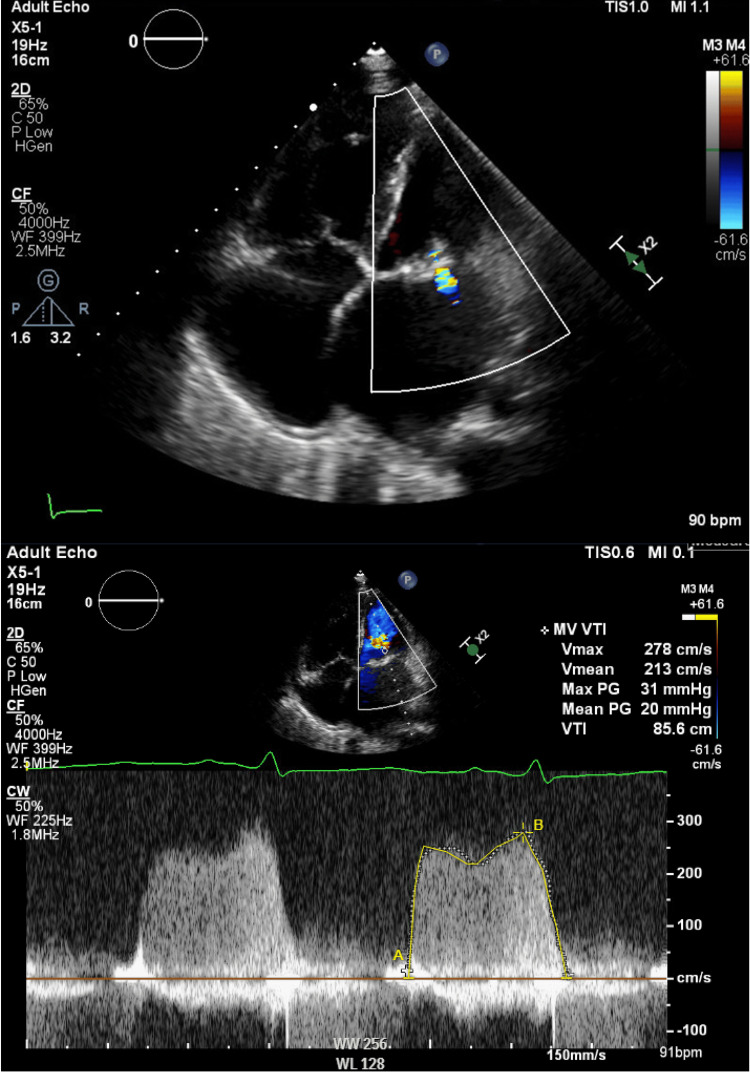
The transthoracic echocardiogram, obtained from the four-chamber apical view Demonstrating severe left atrial dilatation and septal flattening consistent with right ventricular pressure and volume overload. The mitral valve area measured 0.82 cm², with a mean transmitral gradient of 20 mmHg and peak gradient of 31 mmHg, consistent with severe mitral stenosis.
